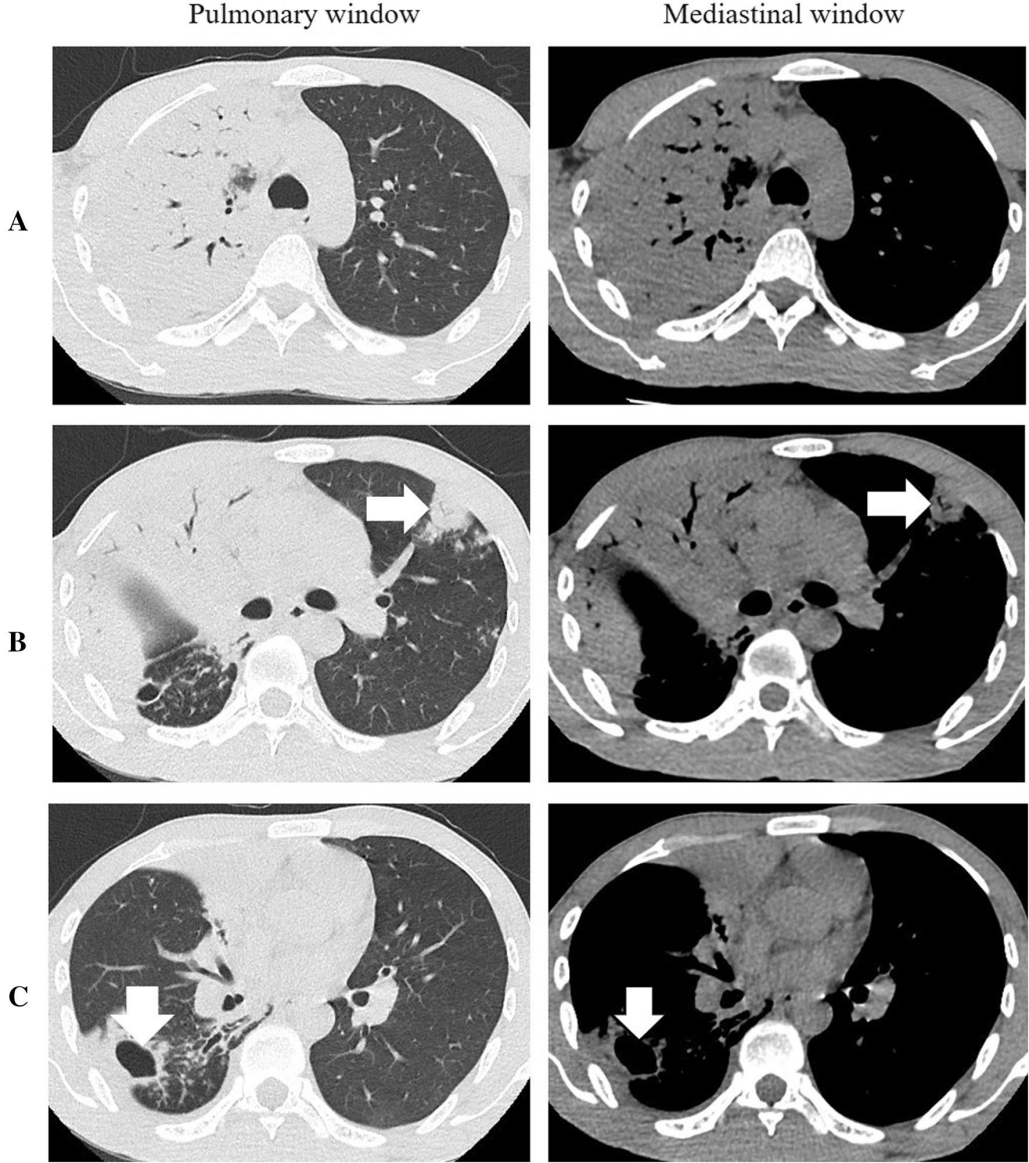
Chest CT confirmed severe pulmonary tuberculosis at admission. Large pieces of density-increased shadow and air bronchogram appeared in the upper right lung (a). The small patch of consolidation in the sub-pleura existed in the left lung, where there were small caves indicated by arrow (b). In the right lower lung lesion, there was a thin-wall cave, as well as pachynsis and adherence of pleura (c). There were neither obvious enlarged lymph nodes in the mediastinum nor the hilar on both sides. The heart shadow was not large, and no effusion or pleural thickening was observed in the chest
